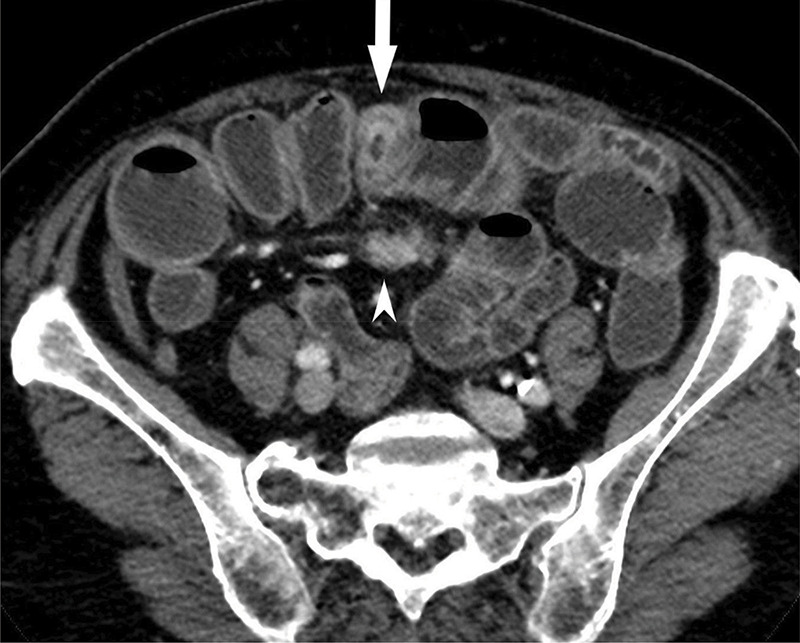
CT showing contrast-enhancing ileal NET (arrow) and mesenteric mass (arrowhead).
